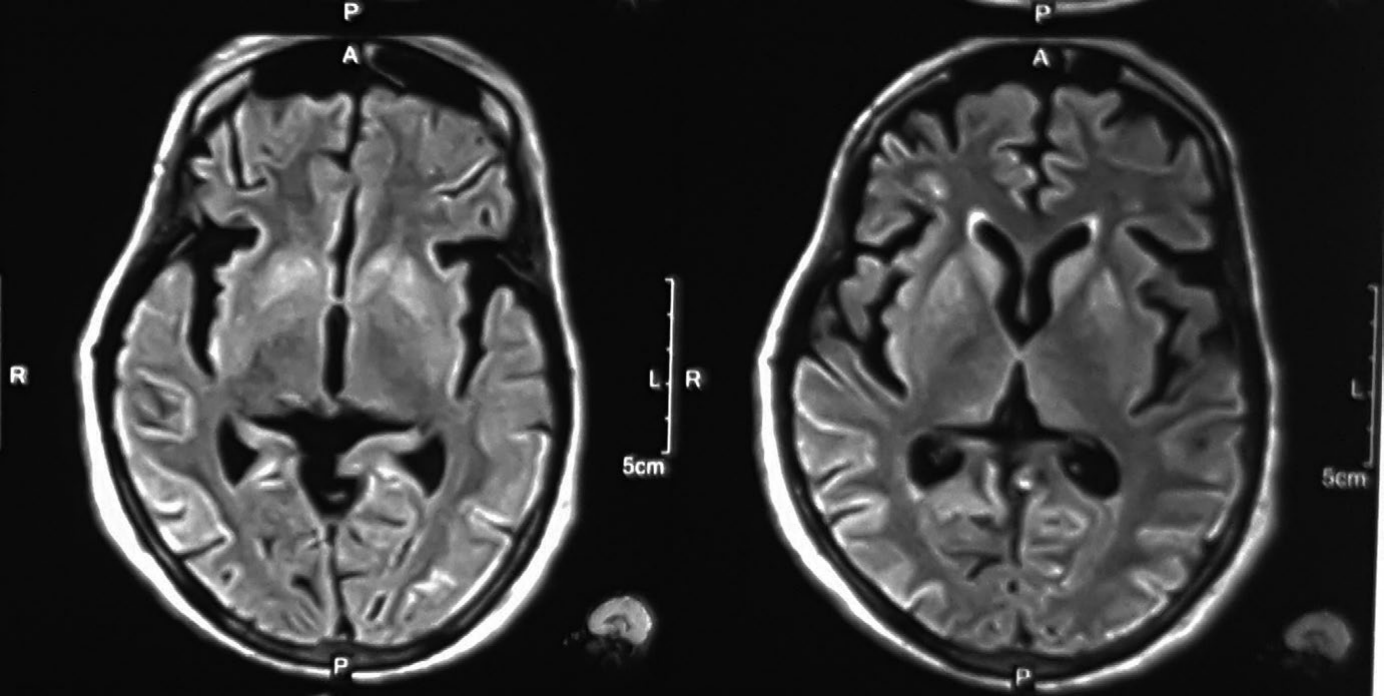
MRI with T2 FLAIR image shows bilateral symmetric basal ganglia hyperintense lesion with cortical ribboning
